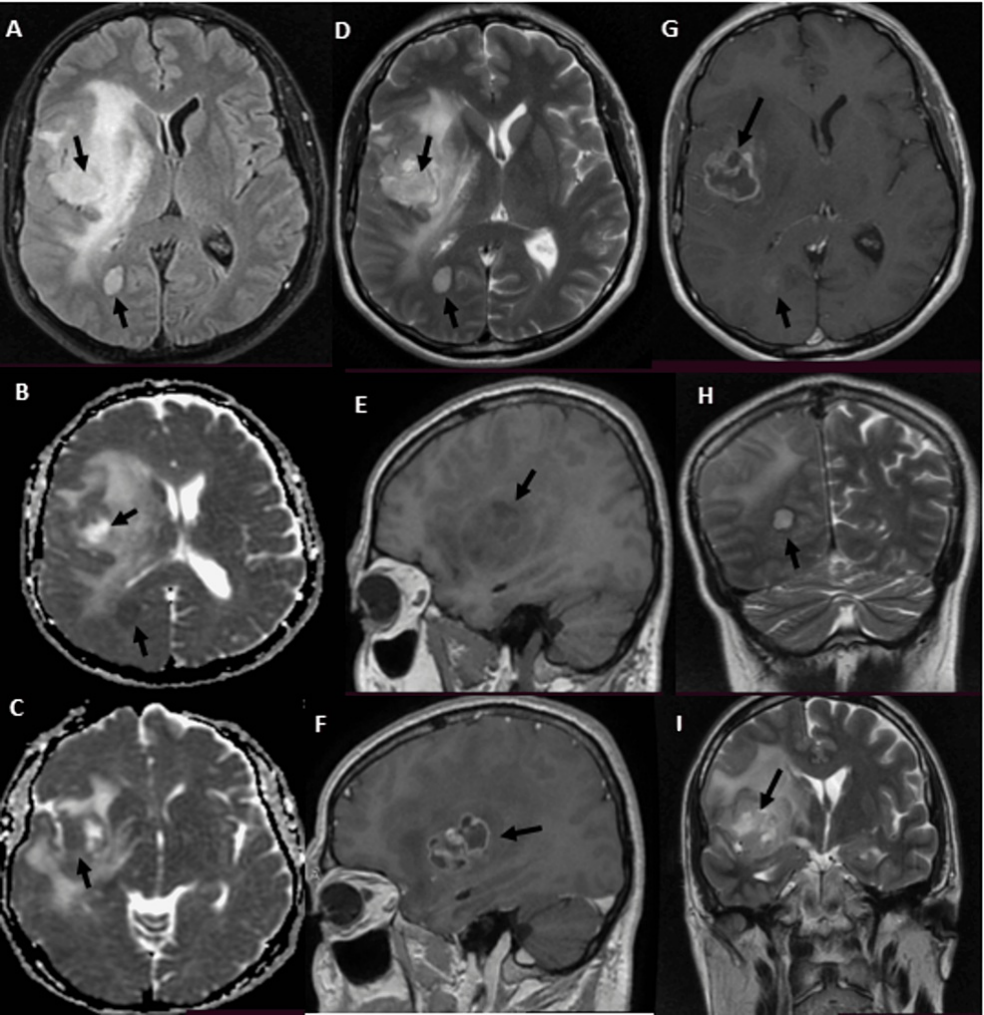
Cranial MRI showing the two lesions, with different contrast uptakes, different FLAIR sequence intensities and different diffuse sequence intensities (A) T1 sequence axial view without contrast; (B, C) DWI axial view; (D) T2 axial view; (E) T1 sequence sagittal view without contrast; (F) T1 sequence sagittal view with contrast; (G) T1 sequence axial view with contrast; (H, I) T2 sequence coronal view FLAIR, fluid-attenuated inversion recovery; DWI, diffusion-weighted; MRI, magnetic resonance imaging Image courtesy: Al Zahraa Hospital University Medical Center (ZHUMC) Radiology Department
